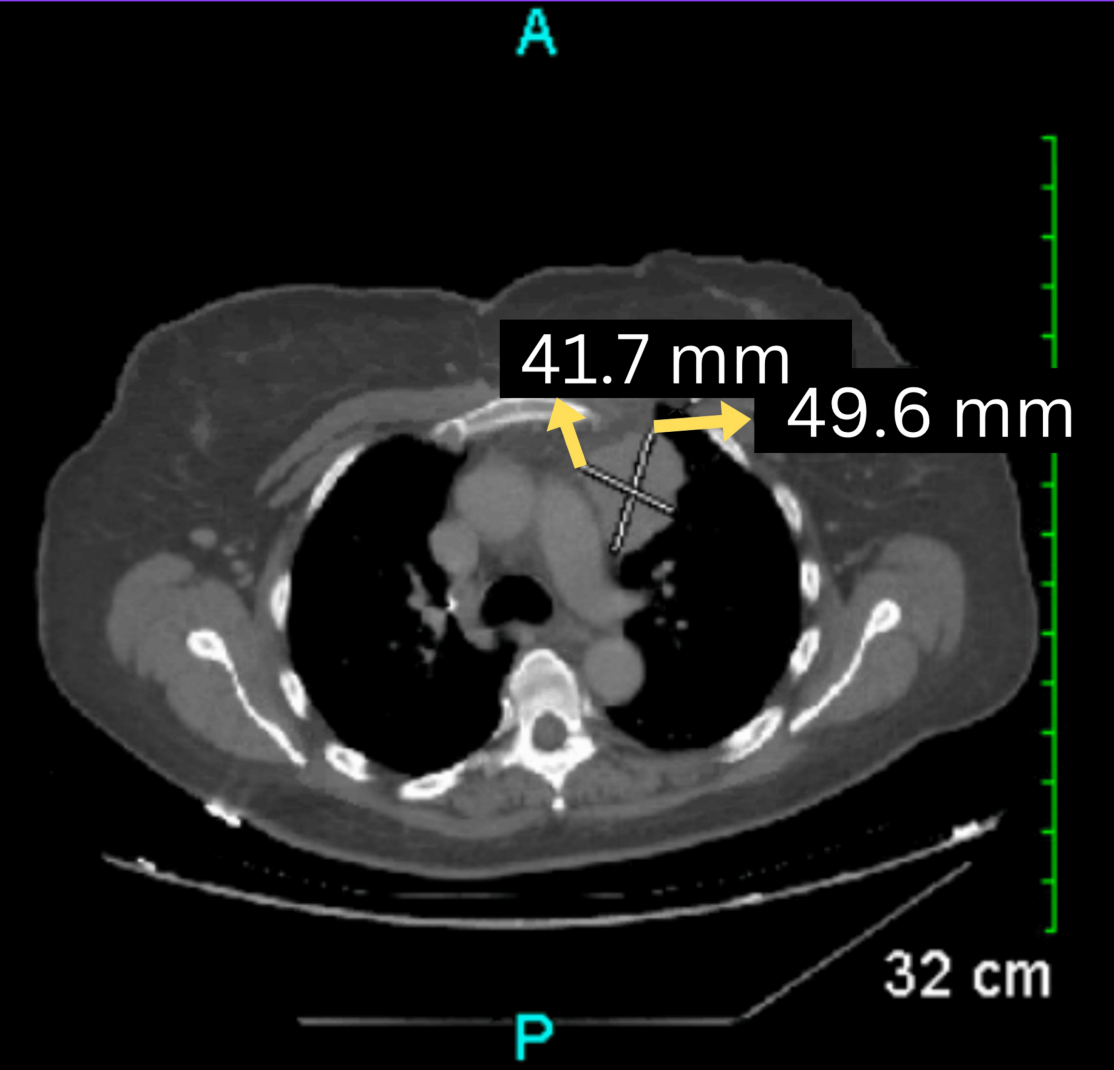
CT scan of the chest revealing an anterior mediastinal soft tissue mass measuring 5.0 x 4.2 cm (yellow arrows), located just anterolateral to the main pulmonary artery and aortic arch. No additional mediastinal or hilar masses were identified. A hiatal hernia is also noted. CT: computed tomography
